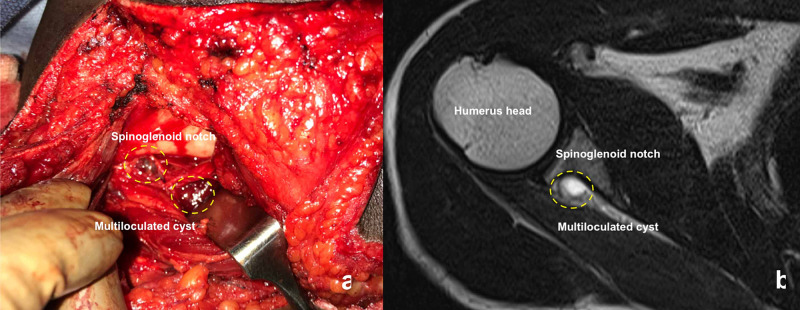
(a) Intraoperative pictures showing the spinoglenoid notch and multiple cysts (yellow dotted circle). (b) MRI images correlating the intraoperative pictures showing the spinoglenoid notch, cysts (yellow dotted circle) and humeral head.
